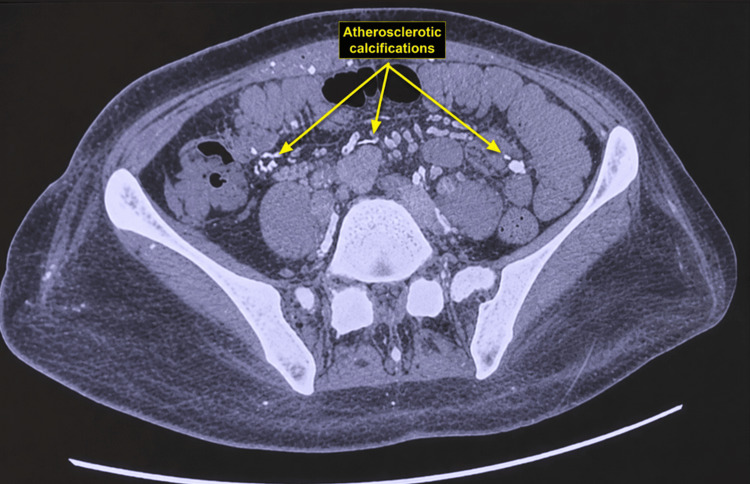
Contrast-enhanced CT abdomen showing chronic atherosclerotic vascular calcifications (arrows) without evidence of active vasculitis CT: computed tomography
